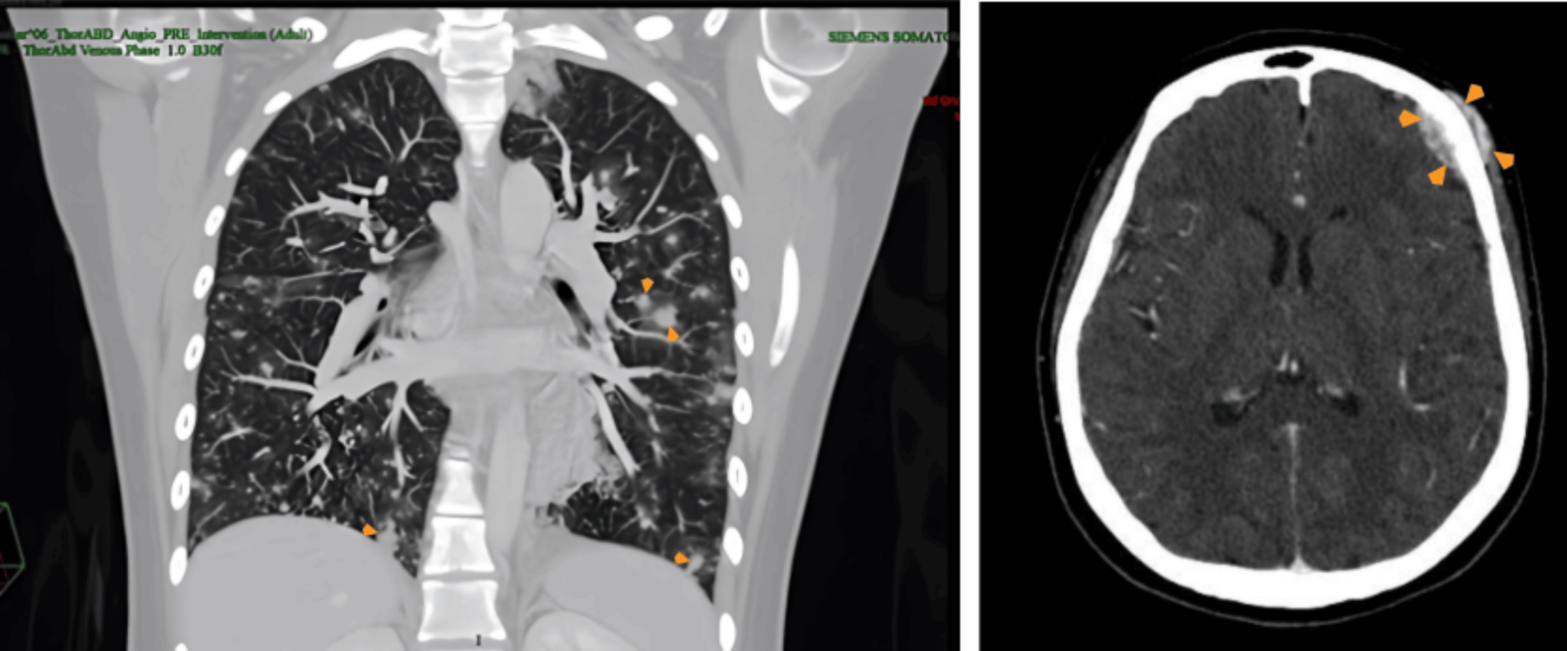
(Left panel) CT scan of lungs and brain on the initial visit with contrast demonstrated multiple nodules in both lungs of different sizes (orange arrowheads). (Right panel) Brain CT scan showing a mass lesion of the skull (orange arrowheads). CT, computed tomography
